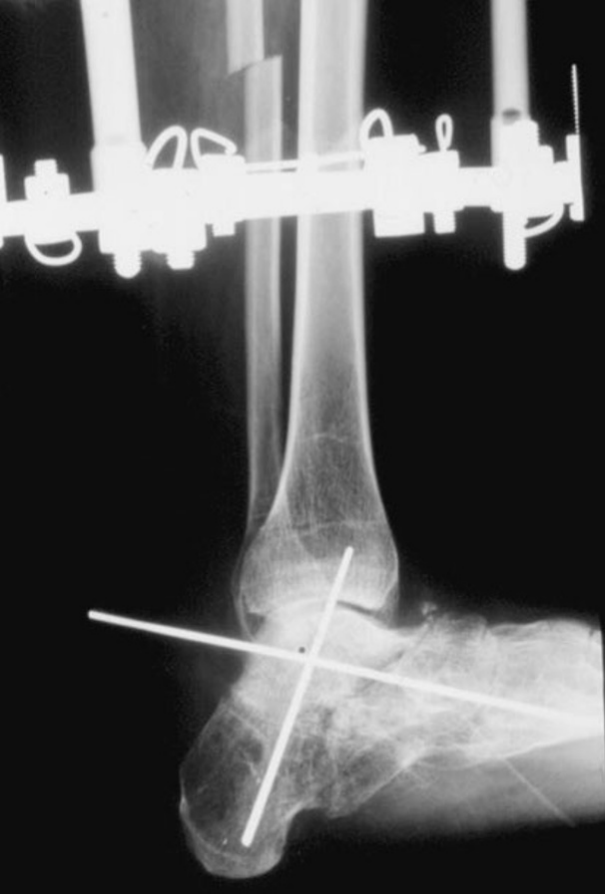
Intraoperative plain X-ray (lateral view) showing *Y-*osteotomy artrorisi with fixation of talus by K-wires
